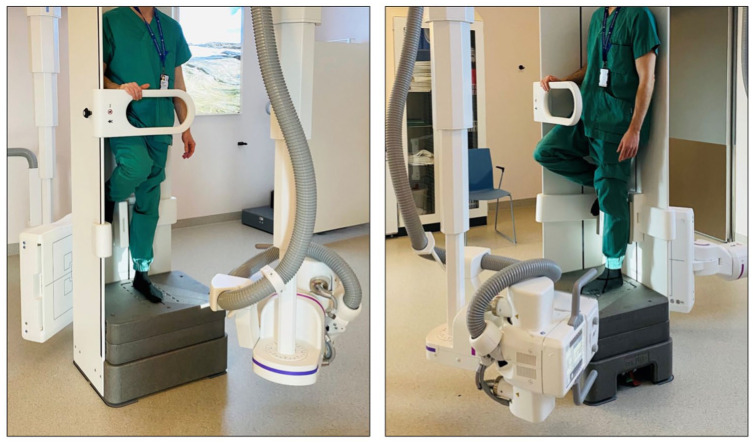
The single-leg stance, weightbearing computed tomography examination was conducted using a twin-robotic X-ray scanner (RAX; Siemens). An identical setup was used for both the injured and healthy foot.
